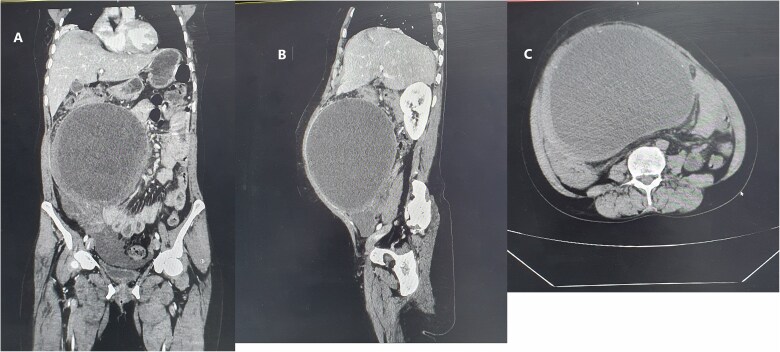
Homogenous hypoattenuating large unilocular cystic mass occupying abdominopelvic cavity with some areas of high attenuating of different viscosity and ovarian vascular pedicle sign as an organ of origin measuring 19.6 × 17.6 × 13 cm (green arrow) (A) coronal enhanced abdominal CT scan (B) enhanced sagittal abdominal CT scan (C) non enhanced upper abdomen axial CT scan.
